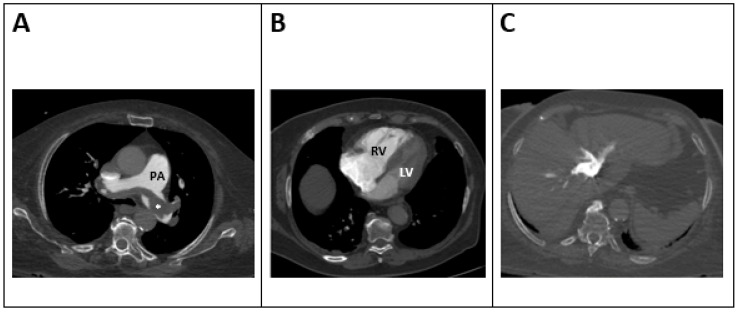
Common finding upon computed tomography in patients with intermediate-risk/submassive PE. (A) The pulmonary artery is shown with a filling defect consistent with a saddle embolus (asterisk). (B) RV dilation in 4-chamber view with an RV/LV diameter ratio > 1. (C) White contrast media is shown within the hepatic veins, demonstrating IVC reflux of contrast to hepatic veins. LV—left ventricle; PA—pulmonary artery; RV—right ventricle. (A) Computed Tomography Angiography demonstrating saddle pulmonary embolus; (B) Computed Tomography Angiography demonstrating right ventricle dilation in 4 chamber views, which looks equal or larger than LV; (C) Computed Tomography Angiography demonstrating inferior vena cava reflux which suggests high right-sided filling pressures.
